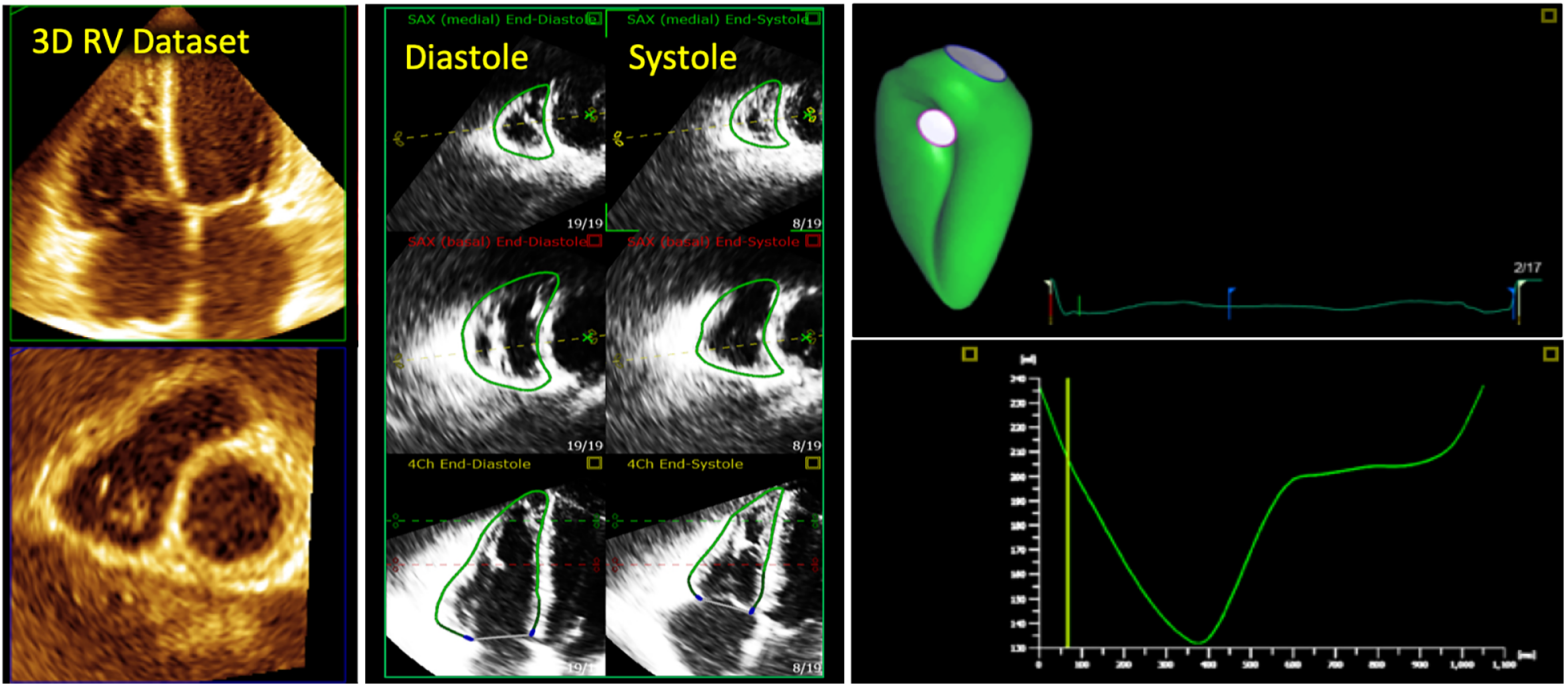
3d echocardiographic analysis of the right ventricle. Far left, 3D dataset of the right ventricle depicting the apical four-chamber and short-axis planes using multi-planar reconstruction. Middle panel shows endocardial tracings overlying the diastolic and systolic phases of the 2D short and long-axis cut-planes obtained from the 3D right ventricular dataset. Far right, top panel shows the 3D endocardial rendered surface while bottom panel shows the volumes obtained after automated software analysis throughout the cardiac cycle.
